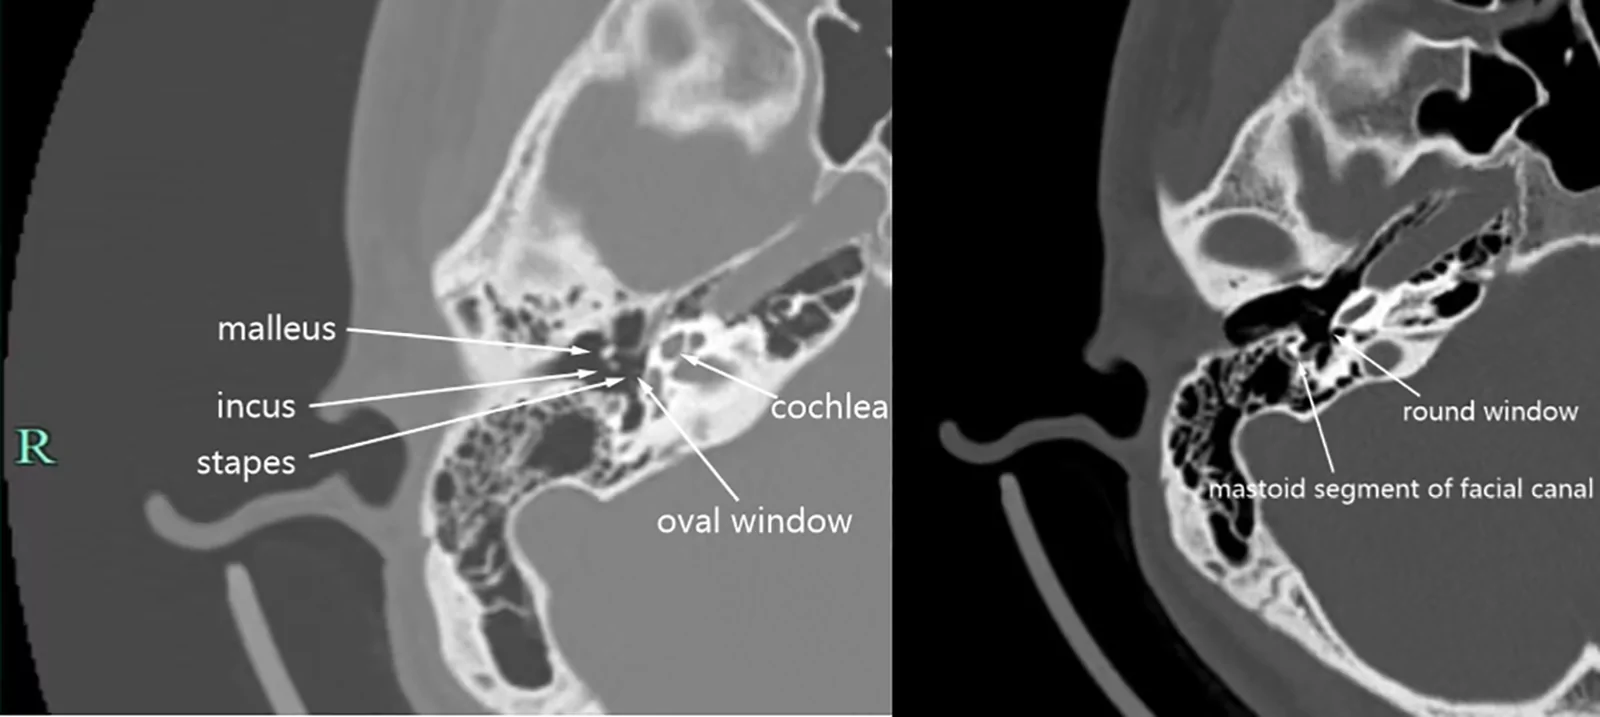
No obvious abnormalities were found on HRCT scan of the mastoid with 3-dimensional. HRCT = high-resolution computed tomography.
